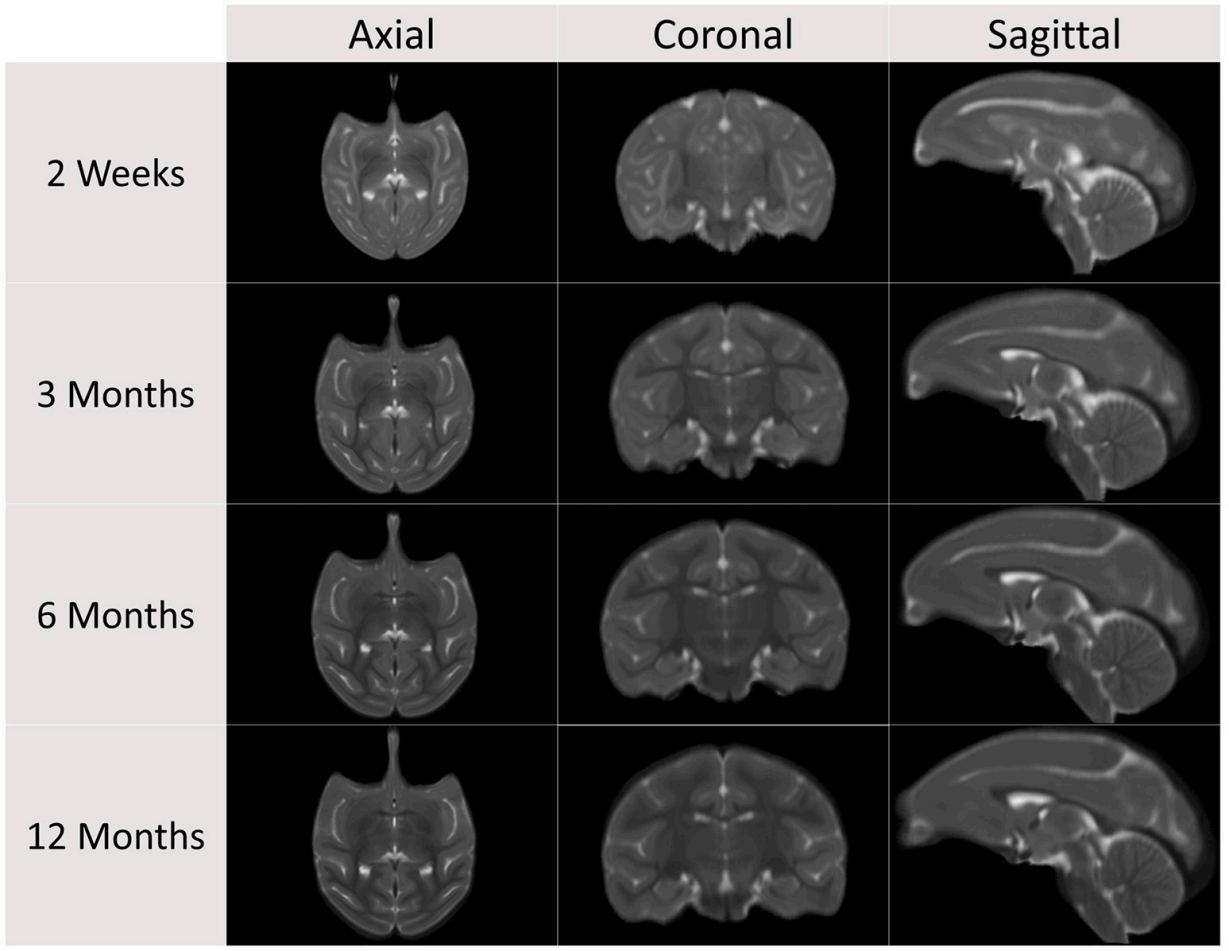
Cross-sectional average T2-weighted atlas images at the four atlas building ages.
